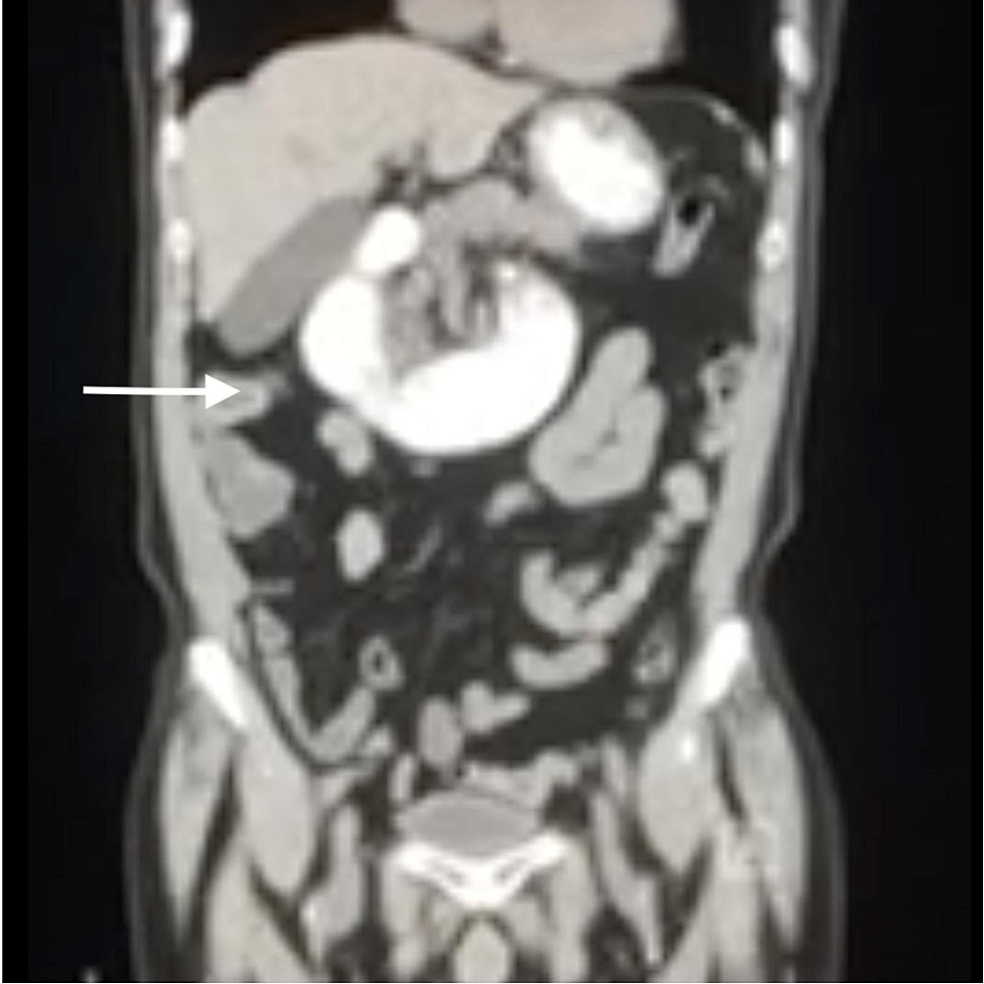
Coronal CT scan of abdomen and pelvis showing the abrupt swirled twisting of small bowel, also called the “coffee bean sign” CT, computed tomography
